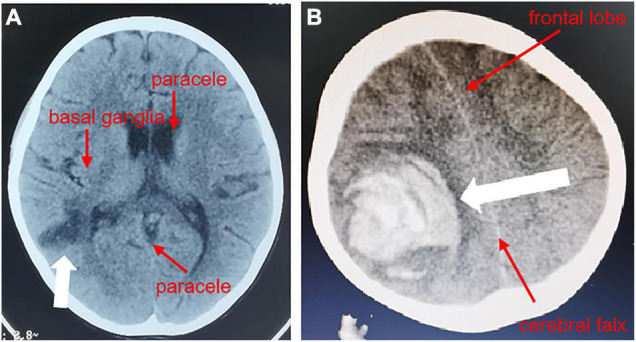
Head CT of the pediatric patient. In the CT results of the head from another hospital, a transverse plane through the inferior portion of the parieto-occipital sulcus showed a hypodense shadow next to the posterior horn of the right ventricle (A), and on the 36th day of hospitalization, a transverse plane through the center of the hemioval showed a right parietal hematoma with herniation of the cerebral sickle (B).
